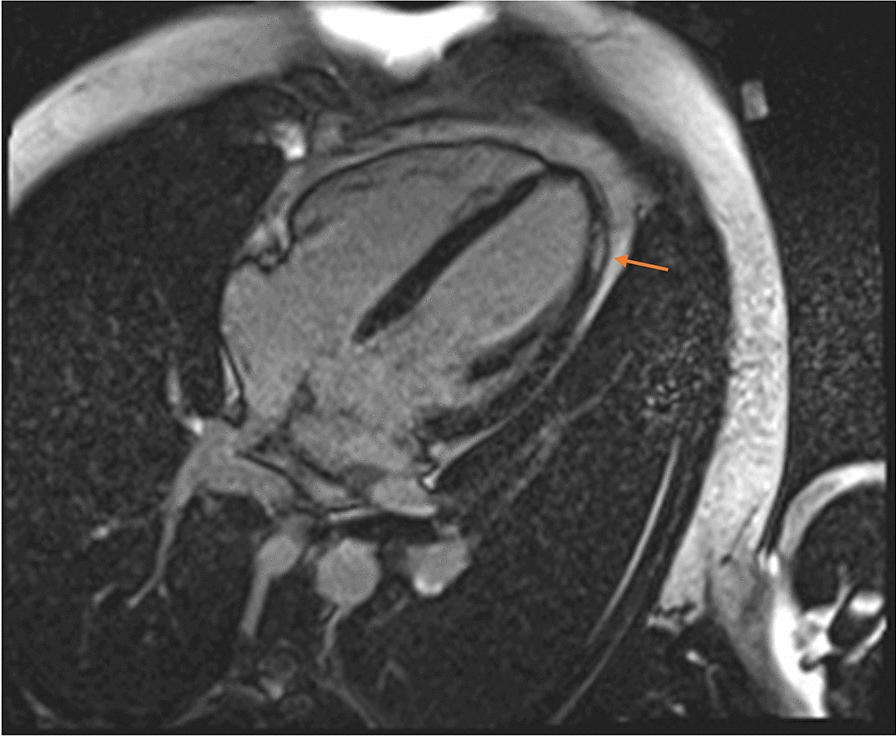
Cardiac magnetic resonance imaging (CMR) showed epicardial late enhancement apical and lateral suggestive of myocarditis. One week post vaccination, the angina was resolved, there were no arrhythmias on Holter monitor, and CK and Troponin returned within normal range. He was discharged on day 7 fully recovered
